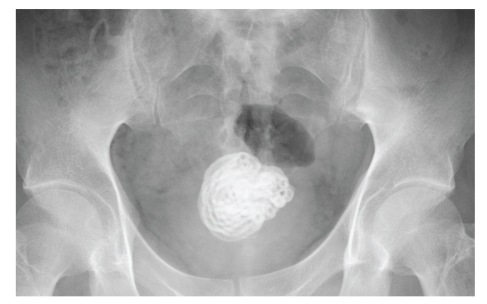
Abdominal plain X-ray showing a convoluted foreign body in the bladder.
